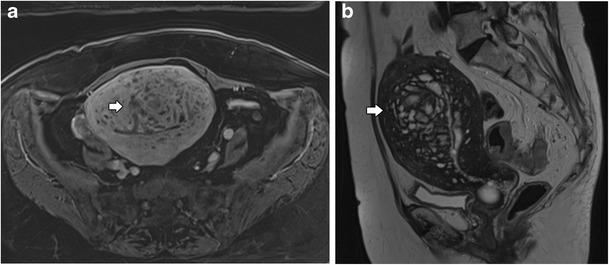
Swiss cheese appearance in adenomyosis: a Axial T1 3D FS- and b Sagittal T2-weighted images; poor definition of the endometrial junctional zone with exuberant glandular myometrial cysts, myometrial nodules and linear striations (white arrows)
